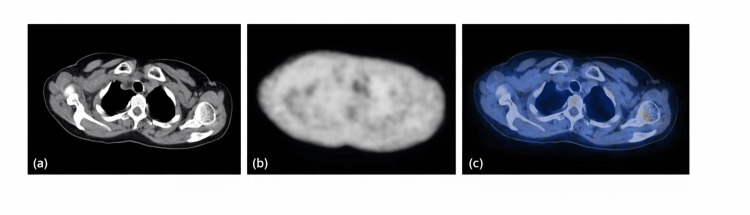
Fluorodeoxyglucose (FDG) PET/CT images at the level of the right subclavian artery. (a) Transaxial computed tomography (CT) image showing the anatomical structures at the thoracic inlet. (b) Corresponding transaxial FDG-PET image demonstrating metabolic activity. (c) Fused transaxial FDG-PET/CT image demonstrating increased FDG uptake along the wall of the right subclavian artery, suggestive of active vascular inflammation.
